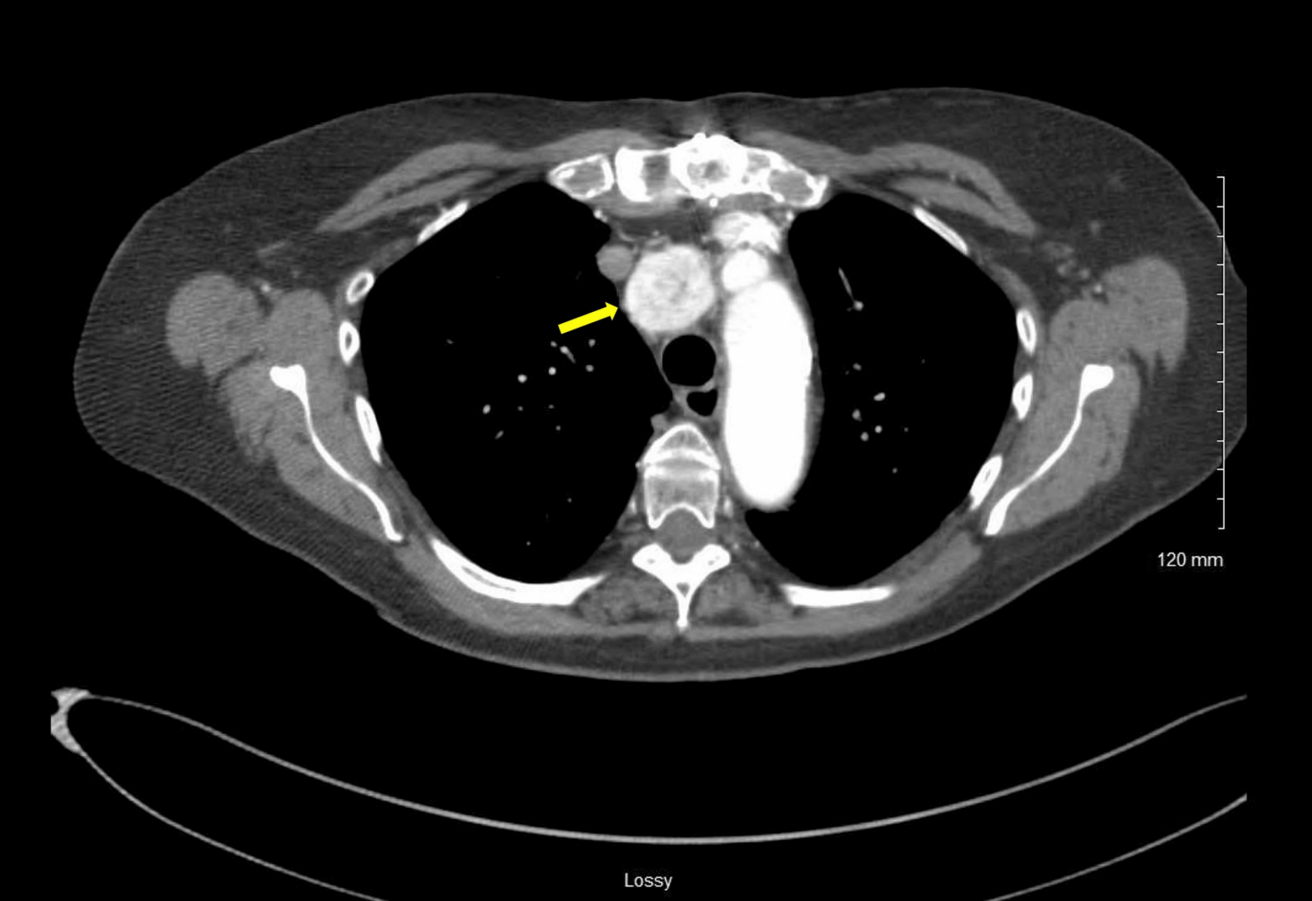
Axial cuts of a preoperative computed tomography of the chest with IV contrast demonstrating a right paratracheal enhancing mass (yellow arrow)
